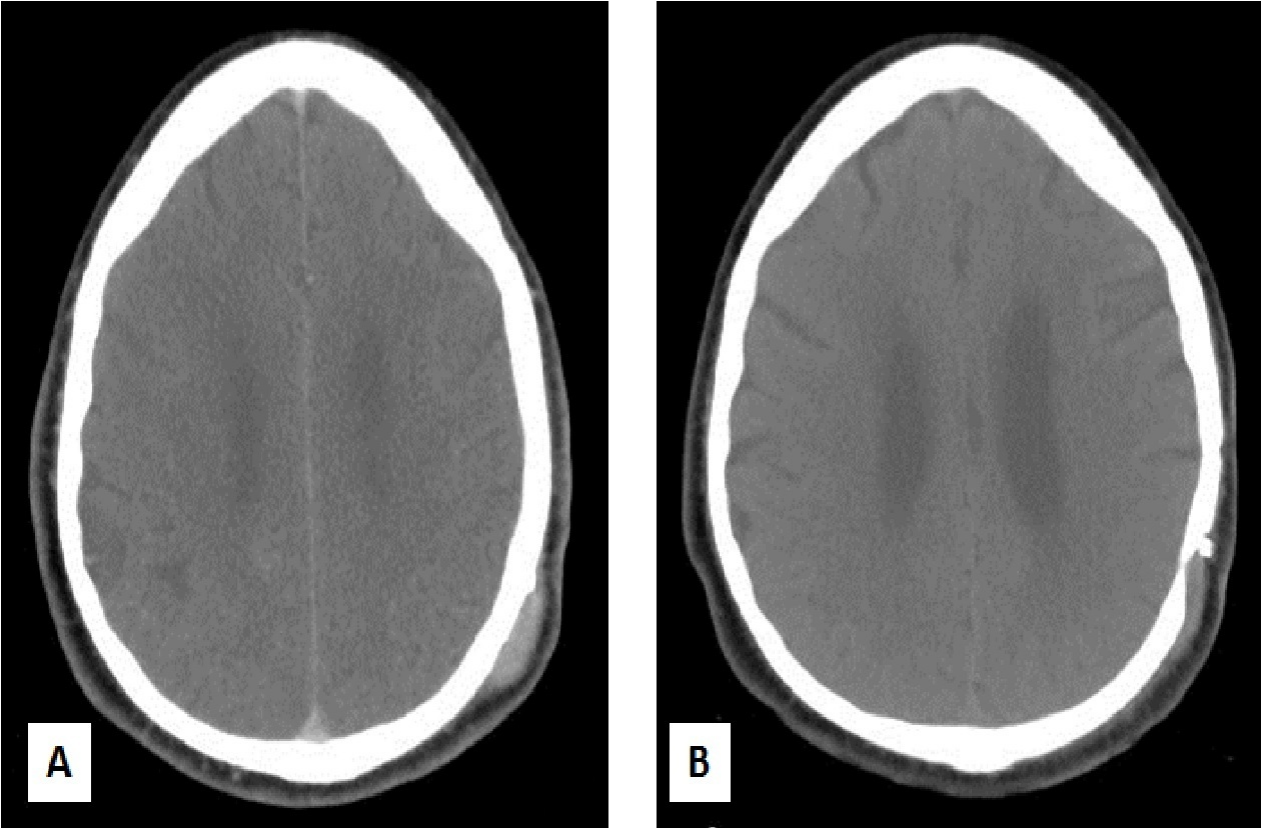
Scalp Metastasis A-B: Axial CT images showing scalp site of metastasis before (A) and after radiotherapy (B). Note the craniotomy defect.
